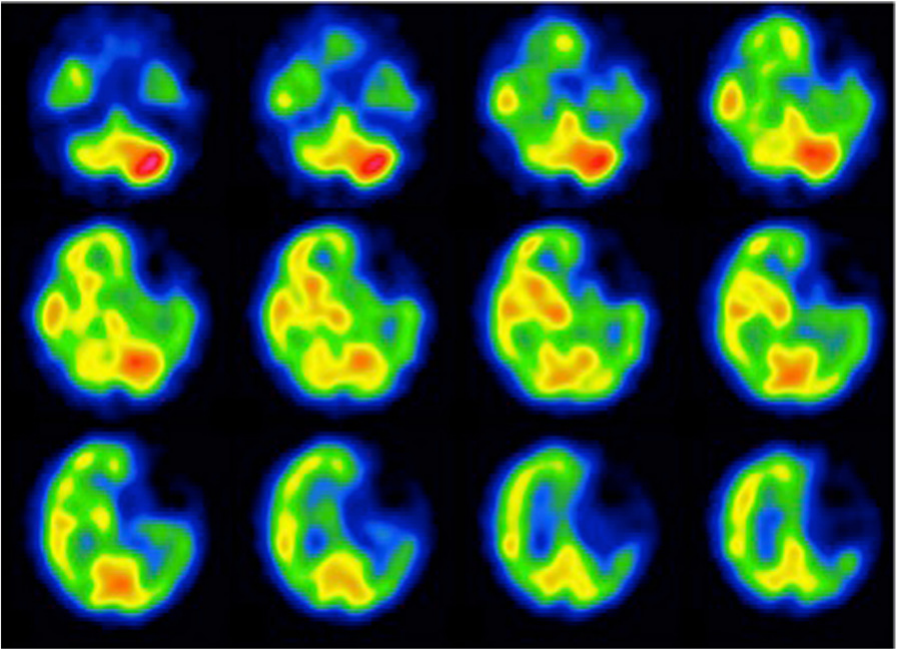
Preoperative single-photon emission computed tomography scan. A preoperative single-photon emission computed tomography scan taken at the regional medical center revealed reduced cerebral blood flow in the left cerebral hemisphere (especially in the external left frontal cortex)
